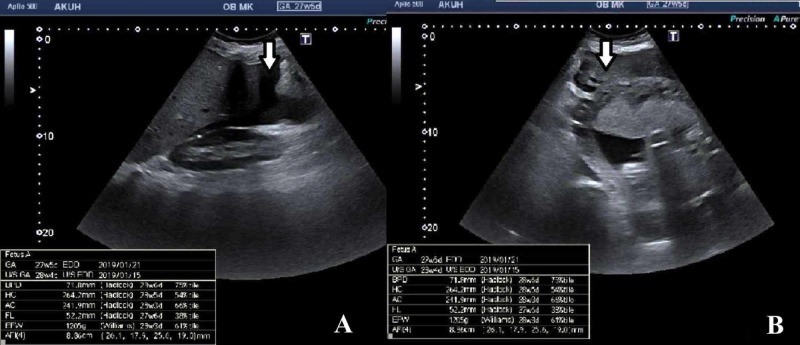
Ultrasound images. Free fluid with internal echoes (arrows) was noted adjacent to Morison's pouch (A) and uterus (B).
